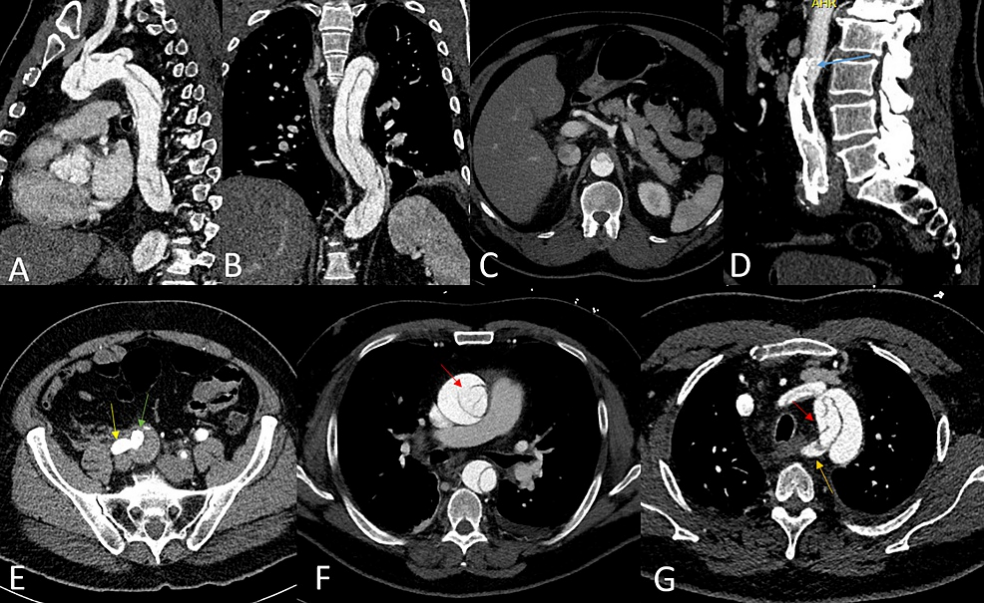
Postoperative Computed Tomography (CT) Imaging An acute aortic dissection extends from the proximal thoracic aorta (A) to the abdominal aorta (B), just below the level of the superior mesenteric artery (C). Dissection flap extends to the bilateral common carotid and subclavian arteries. The proximal end of the endograft was partially collapsed due to the dissection (D). No endoleak or dissection was noted in the right iliac artery aneurysm (E). Proximal ascending aorta (F) and right subclavian origin (G) entry tears seemed to correspond to intraoperative findings. Red arrows: entry tears. Yellow arrow: external iliac artery. Green arrow: internal iliac artery. Blue arrow: collapsed proximal endograft. Orange arrow: aberrant right subclavian artery. (A) Sagittal thoracic CT image. (B) Coronal thoracic and abdominal CT image. (C) Axial abdominal CT image. (D) Sagittal lumbar CT image. (E) Axial abdominal CT image. (F,G) Axial thoracic CT image.
